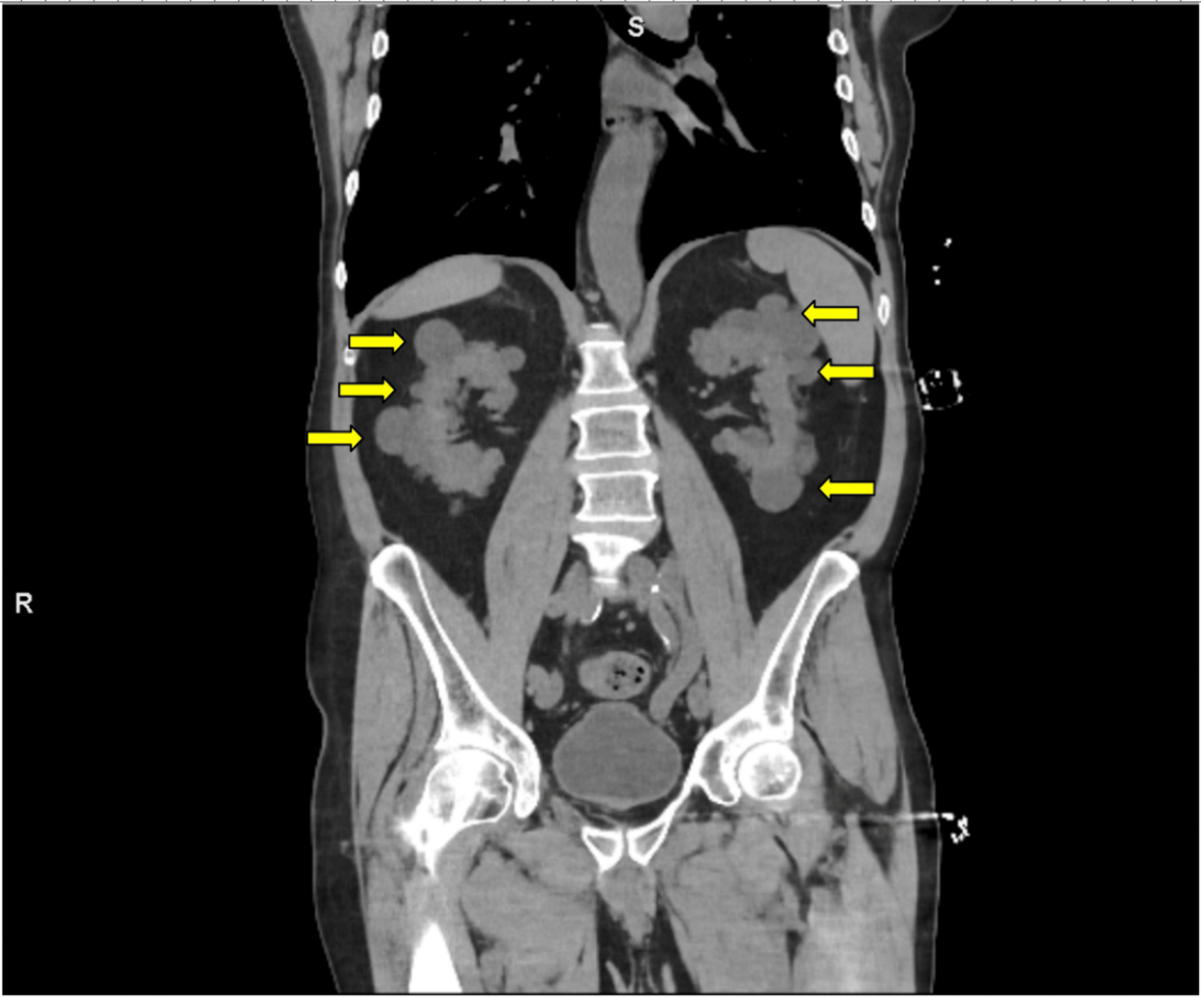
Coronal abdominal and pelvic CT scan W/O contrast showing numerous bilateral renal parenchymal cysts indicated by arrows
